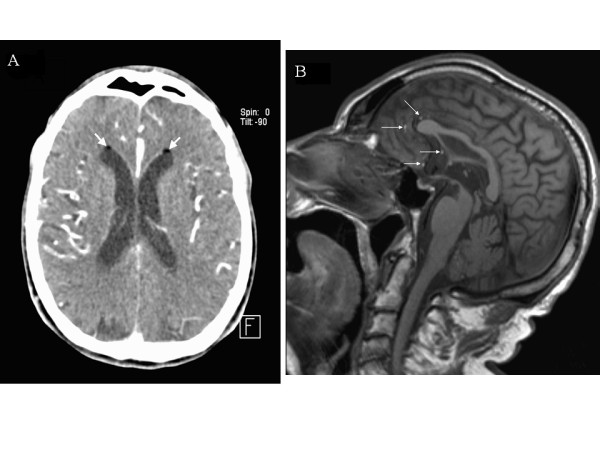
Head CT-scan and MRI image. A. Post contrast head CT-scan: fat droplets in the frontal horns of the lateral ventricles (white arrows). B. Sagittal *T_1_*-weighted head MR image: fat droplets disseminated in the subarachnoid spaces (white arrows).
